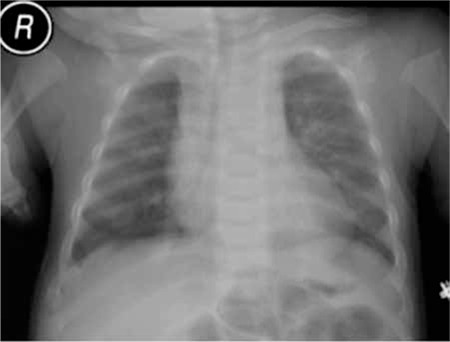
Case 2- chest X-ray demonstrating costo-chondral widening
